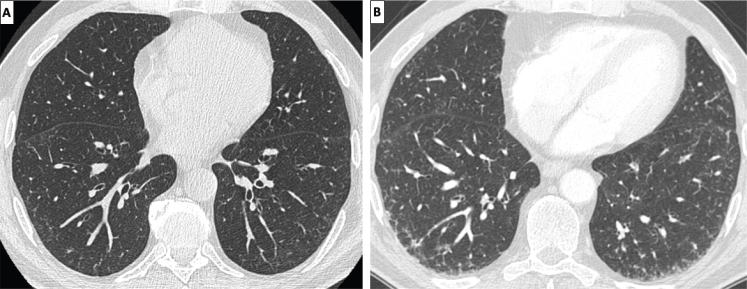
Example of chest HRCT images reconstructed prior (A) and after (B) contrast medium administration, demonstrating a generalized increase of lung density in B as compared to A. HRCT, high resolution computed tomography.
